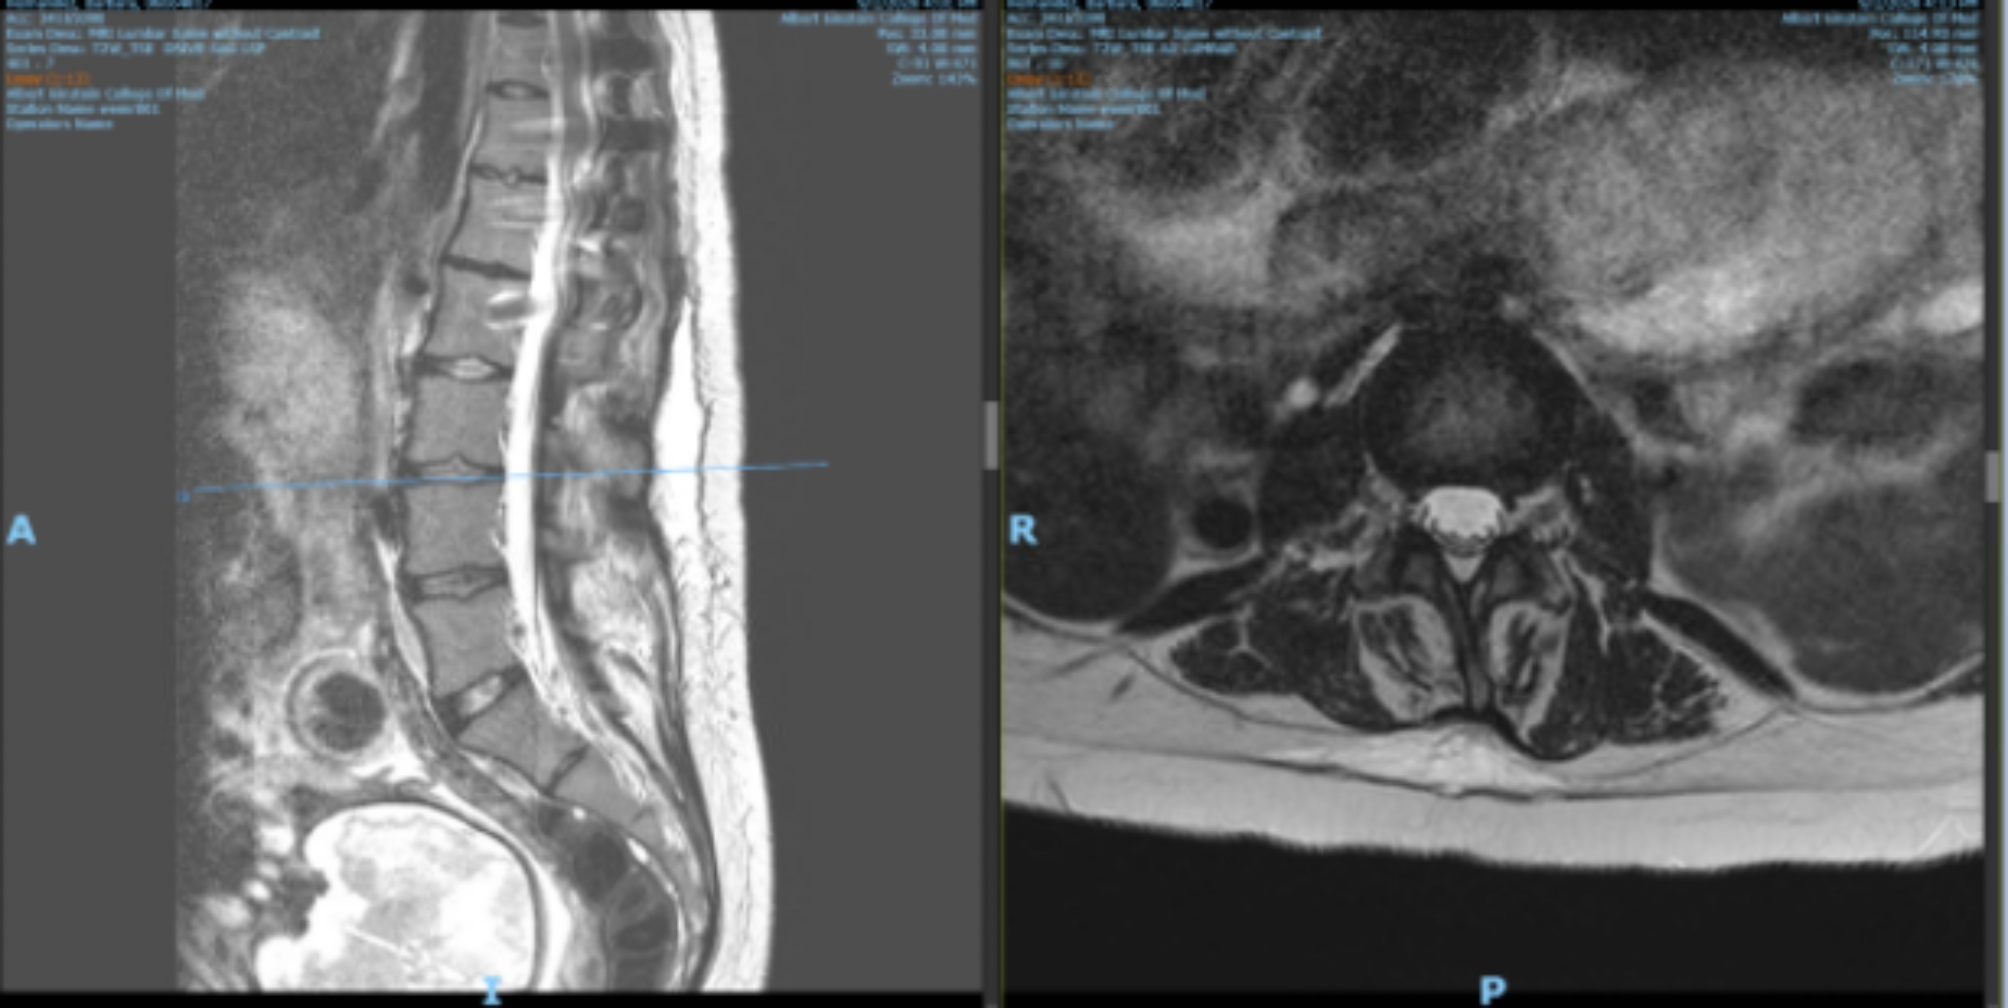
MRI of the lumbar spine confirmed the presence of surgical rods
